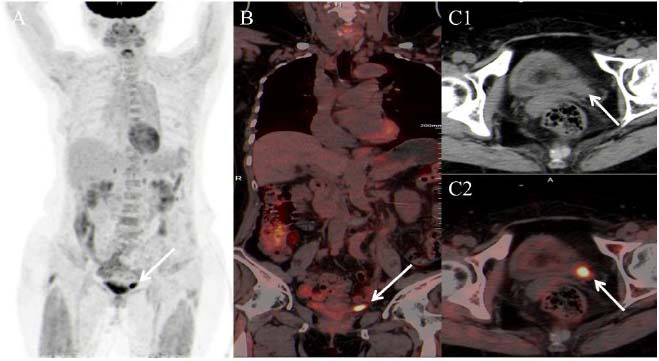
The lesion shows clearly with intensive metabolic activity (SUVmax= 12.64) on ^18^F-FDG PET/CT scan (arrows). (A) Maximum intensity projection image; (B) Coronal fusion image; (C1) Transverse CT plain scan image; (C2) Transverse fusion image).
